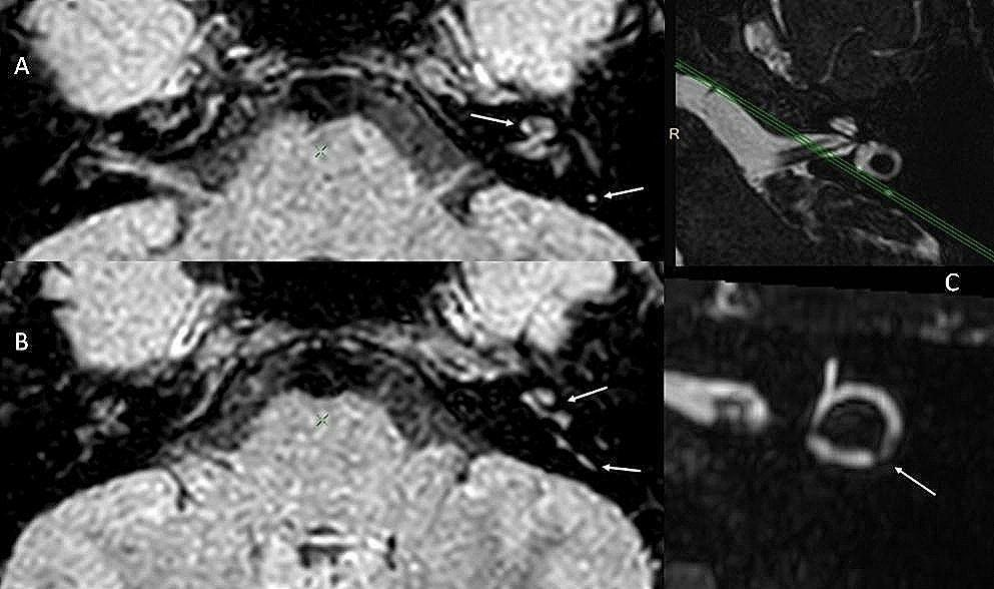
1.5 Tesla MRI performed 4 h post-intravenous gadolinium injection revealed hyperintensity within the left cochlea and posterior semicircular canal (arrows) on 3D FLAIR imaging (A) (B), and a minor fluid amputation from the left posterior semicircular canal (arrow) visualized on 3D DRIVE imaging reconstruction in the axis of the canal (C)
